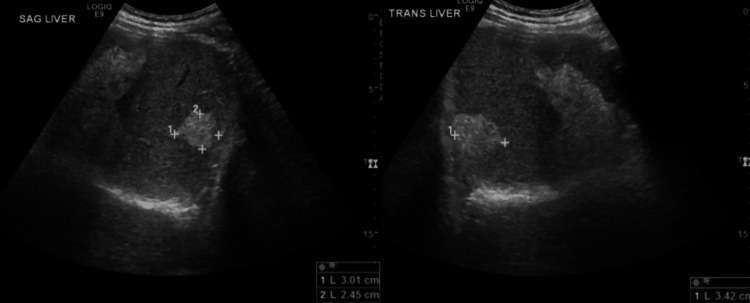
Serial liver ultrasound after discharge Multiple hyper-echogenicities up to 5 cm throughout the liver.
